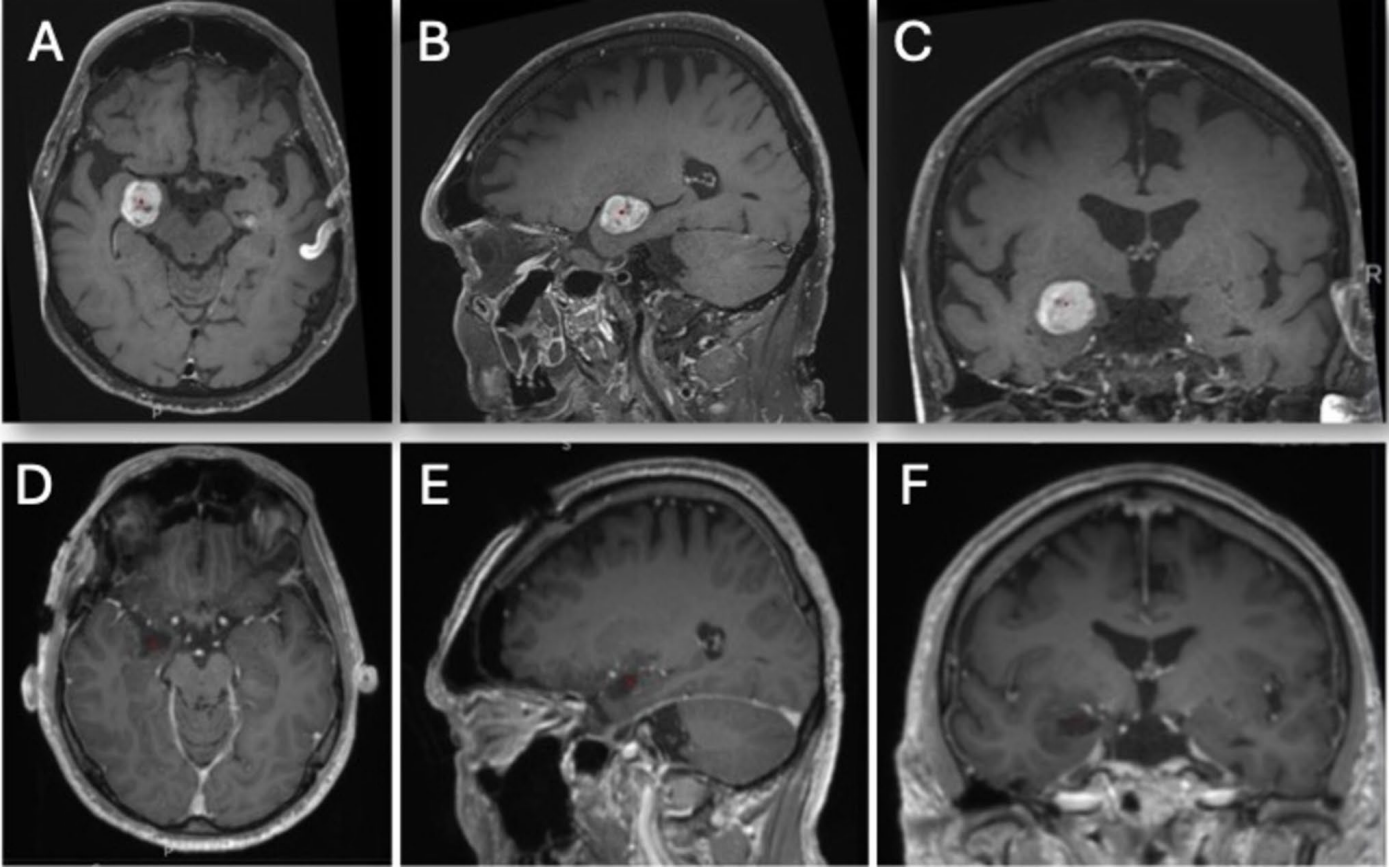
A–C Preoperative gadolinium-enhanced T1-weighted axial, sagittal, and coronal MR images depicting a well-defined, round, enhancing lesion within the amygdala. D–F Postoperative gadolinium-enhanced T1-weighted axial, sagittal, and coronal MR images demonstrating gross total resection with no evidence of neocortical disruption
